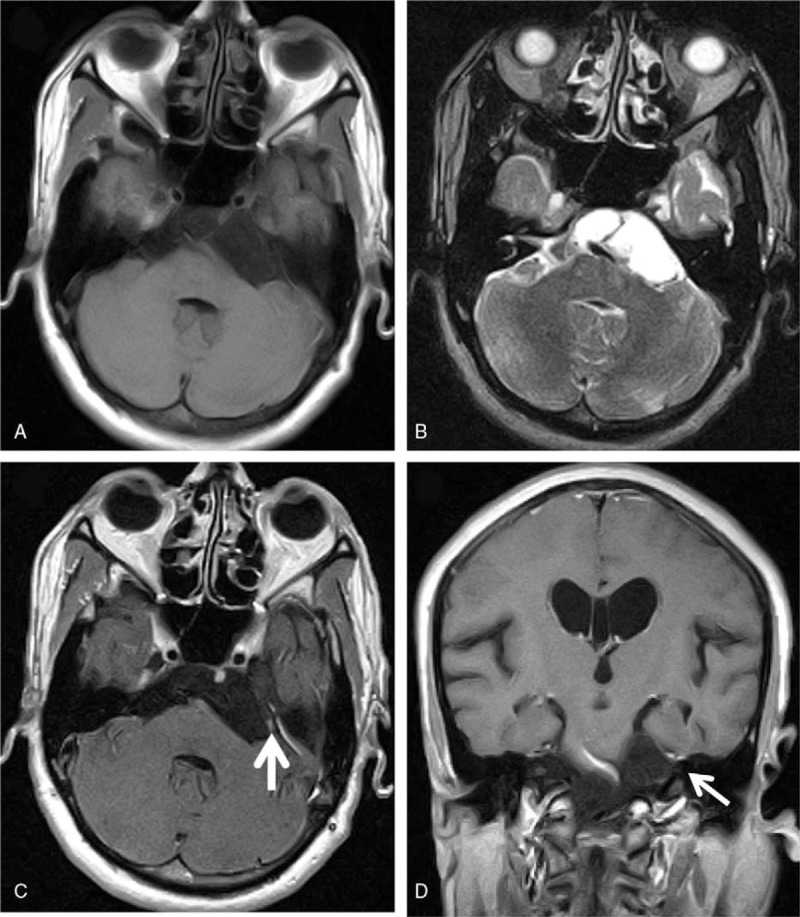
Preoperative MRIs show a cyst-like lesion in the left cerebellopontine and prepontine cistern on (A) T1-weighted axial image, and (B) T2-weighted axial image. On contrast enhanced (C) axial and (D) coronal images, the lesion shows a faint enhancement in its lateral portion (arrows). MRI = magnetic resonance imaging.
